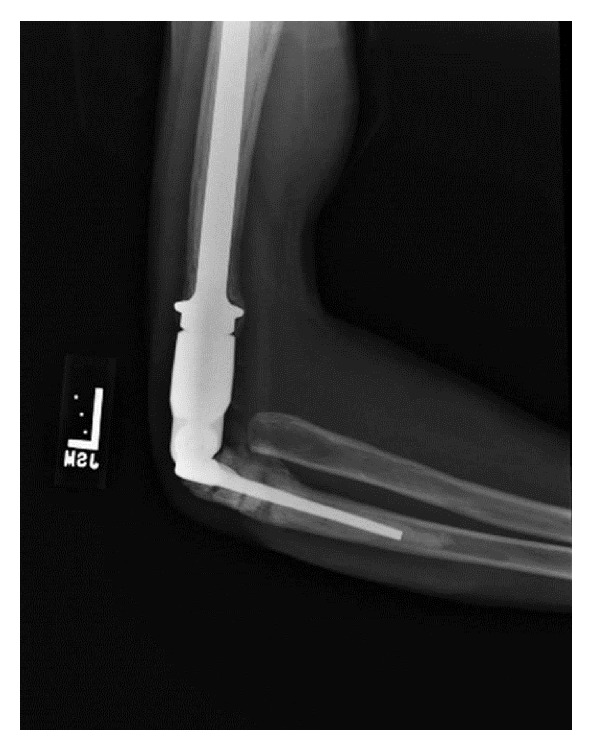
Plain radiograph taken at the patient's 4.5-month appointment reveals a healed ulna nonunion and a stable total elbow prosthesis without signs of loosening.
